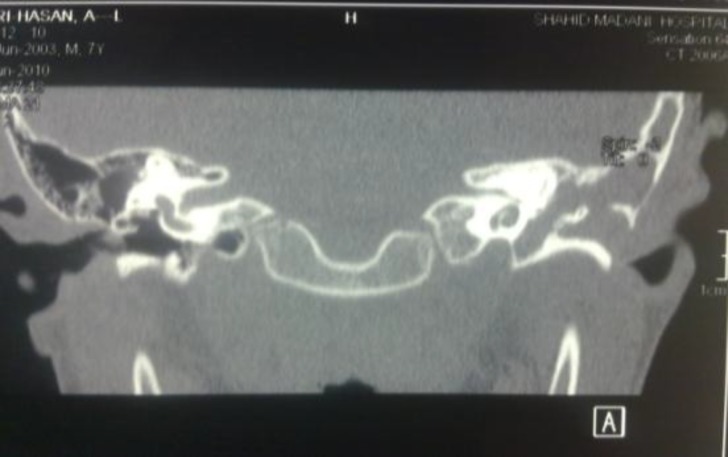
Computed tomography (CT) scan from first branchial cleft fistula opening in the middle ear showing automastoidectomy and the appearance of soft tissue in the middle ear and mastoid
